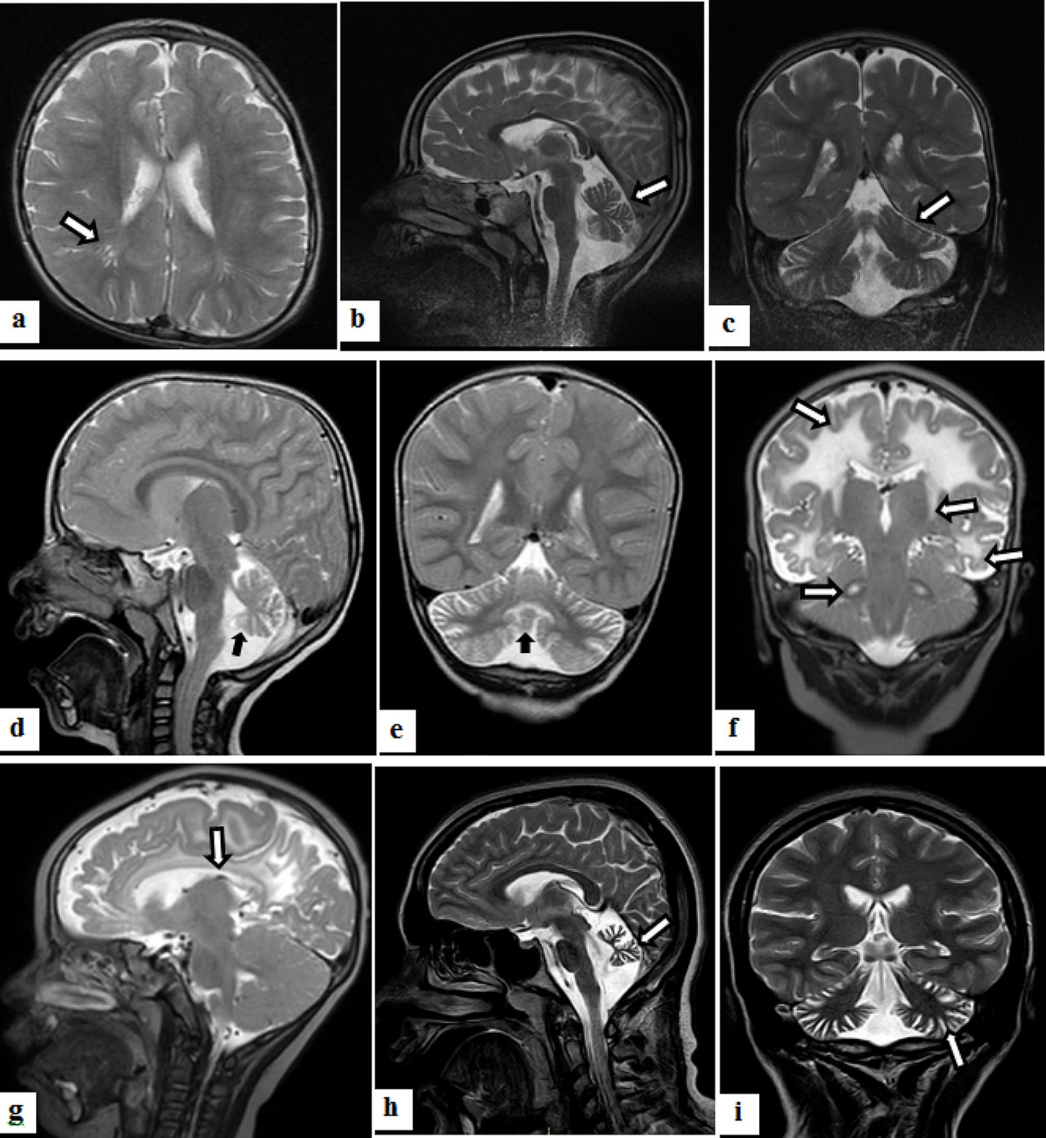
MRI of patients with c.574T > C (*CWF19L1*) variant (a-c), c.388G > A (*THG1L*) variant (d,e), c.1781G > A (*DARS2*) variant (f, g) and c.398 A > G (*BRAT1*); (a) Axial T2-Weighted Brain MRI shows increased peri-vascular spaces. (b) Sagittal and (c) Coronal T2- Weighted Imaging shows cerebellar (prominently vermis) atrophy. d and e) T2-weighted sagittal and coronal images showing cerebellar atrophy. f) Coronal T2-Weighted Brain MRI is indicative of white matter, internal capsule and bilateral dentate involvement. g) Sagittal T2-Weighted view shows corpus callosum atrophy. h) Sagittal and i) Coronal T2-Weighted Brain MRI shows severe cerebellar atrophy
